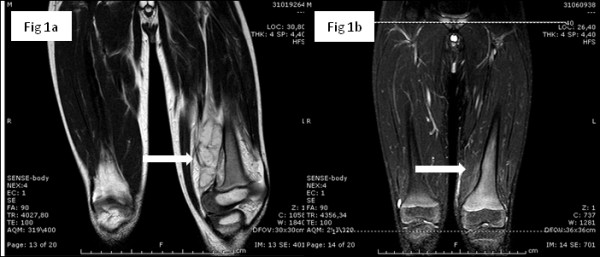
MRI of the left knee prior and post curettage and antifungal therapy. Figure 1a: voluminous neoformation (arrow) with expansive character, inhomogeneous signal intensity and polycyclic contours, extending longitudinally for about 9 cm, infiltrating the soft tissues adjacent to the distal diaphysis and the metaphysis of the femur on the left. The mass has multiple cistic-like areas compatible with signs of intralesional necrosis and determines colliquative loosening and dislocation of the periosteal membrane that appears broadly interrupted. No significant erosion of the cortical bone and cancellous bone adjacent to where we except a focal thinning of the cortex. No injury to the remaining of femoral bone. The muscular structures, in particular the vastus medialis muscle and the biceps femoris, have altered perilesional signal intensity and appear displaced and compressed, without a safe plane of cleavage with the injury. Figure
